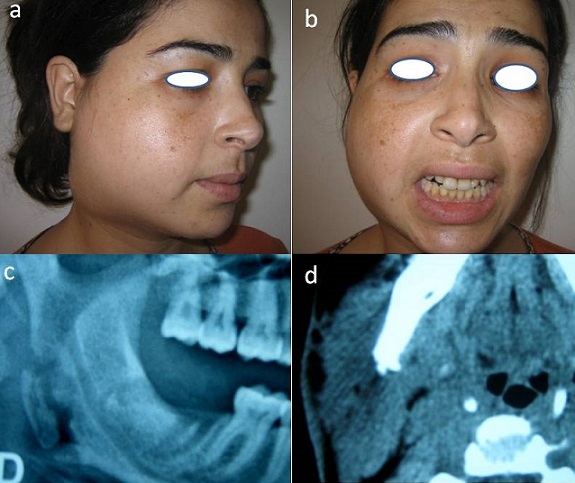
a) phlegmon de la loge massétro-parotidienne droite; b) trismus serré; c) ostéite du ramus avec séquestre osseux sur l'orthopantomogramme; d) aspect scanographique (en coupe axiale) avec infiltration cellulitique de la loge masséterique
